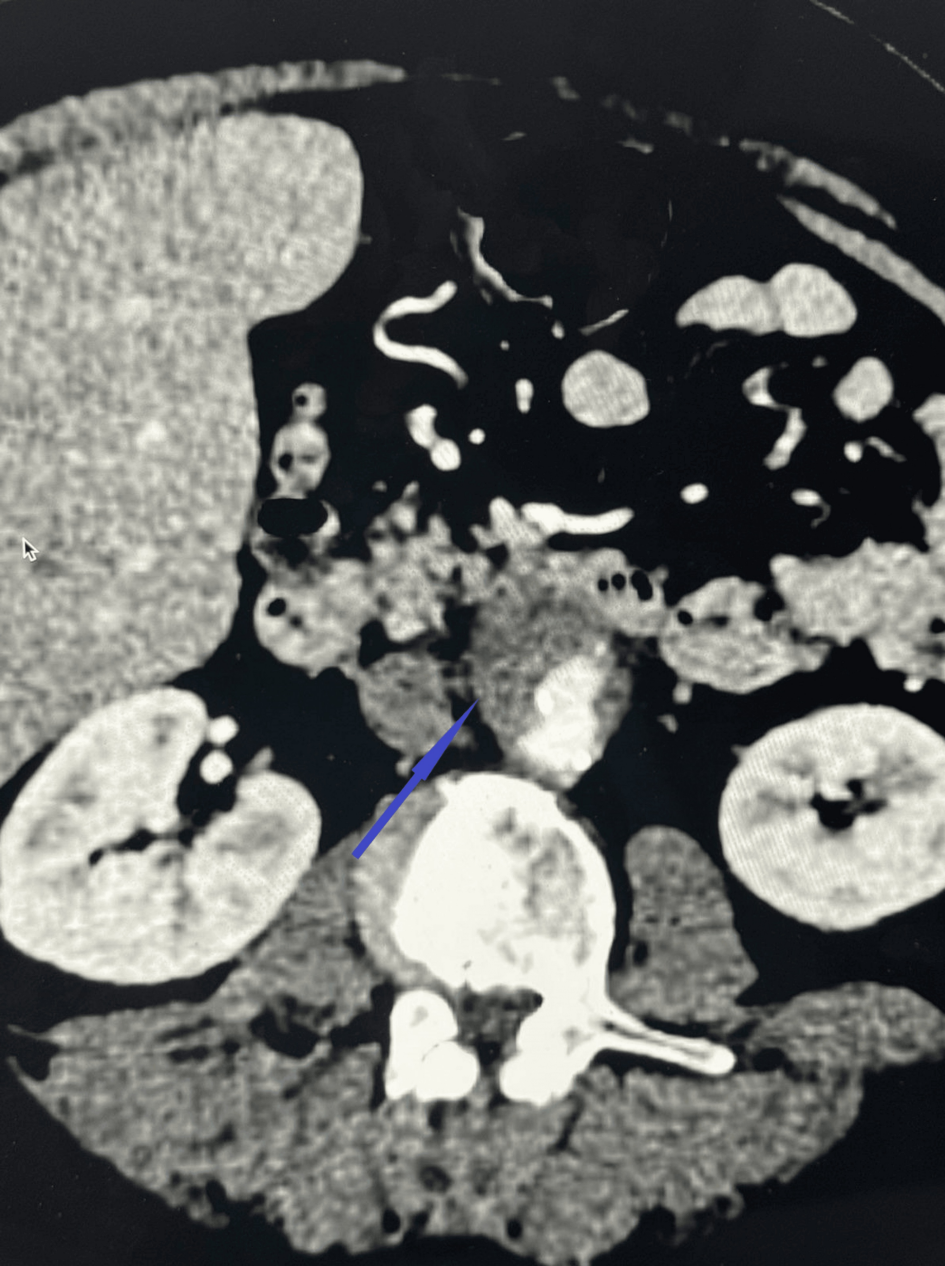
Abdominal computed tomography angiography (CTA) Abdominal computed tomography angiography (CTA) demonstrating changes in the distal abdominal aorta most consistent with a penetrating atherosclerotic ulcer (blue arrow). These findings are accompanied by infiltration of the perivascular adipose tissue, extending paraaortically and involving the level of the bilateral common iliac arteries.
